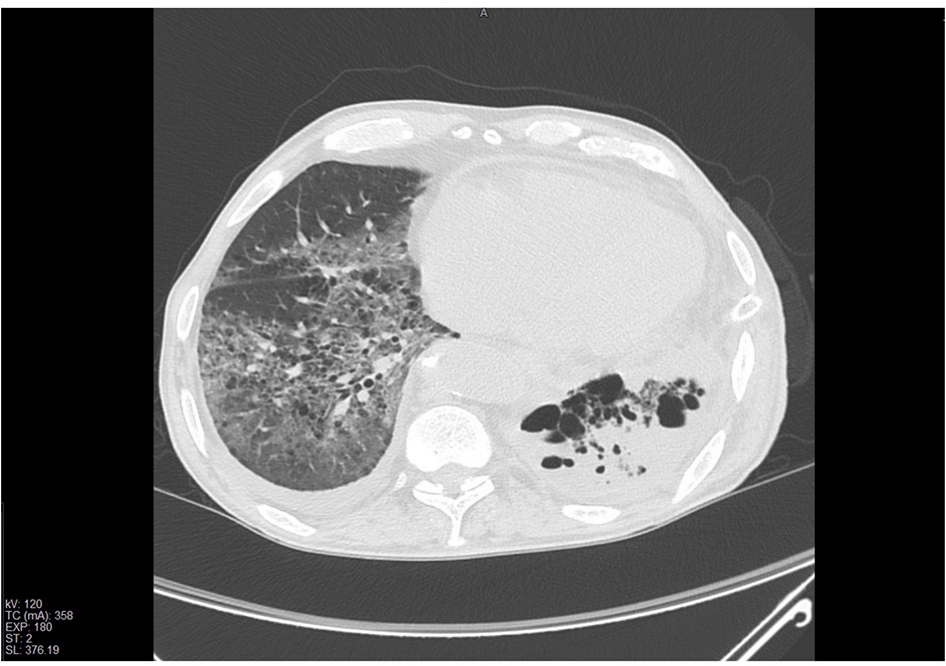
Chest CT-scan showing the presence of extensive pulmonary infarction in the residual parenchyma.
